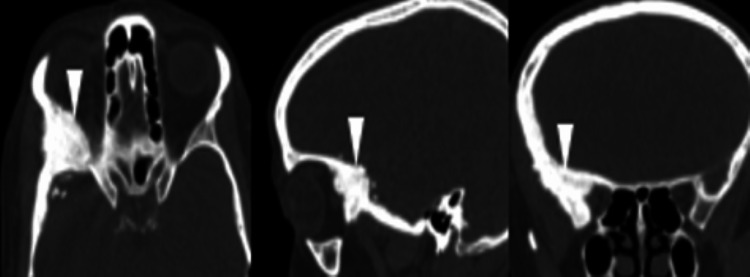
Brain scan Cerebral CT bone window in axial, sagittal, and coronal sections: spheno-orbital osteocondensing lesion with loss of right cotico-spongiosa differentiation.
